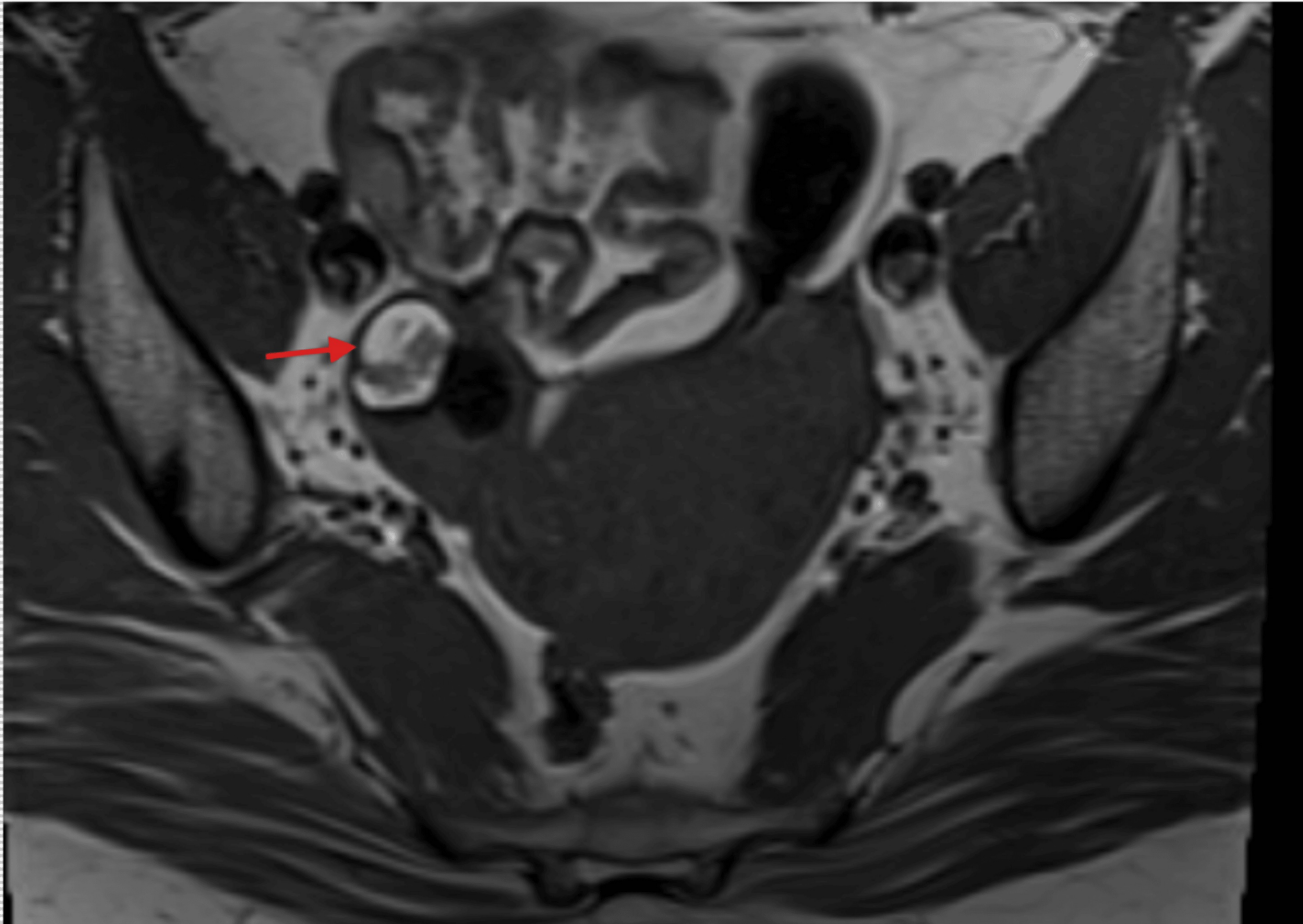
Case 2: MRI of the pelvis (red arrow: dermoid cyst in the right adnexa)
